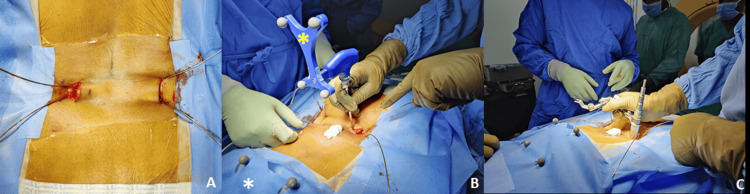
(A) Intraoperative image of an L4-5 MISS-TLIF, showing guidewires placed in a convergent manner using 3D CT O-arm navigation. (B) A medialized fascial incision was made through the same 1-inch skin incision, and a navigated PAK needle was employed to determine the precise trajectory (yellow asterisk) for decompression and fusion. (C) The tubular retractor was then docked onto the predetermined, navigation-guided guidewire.
